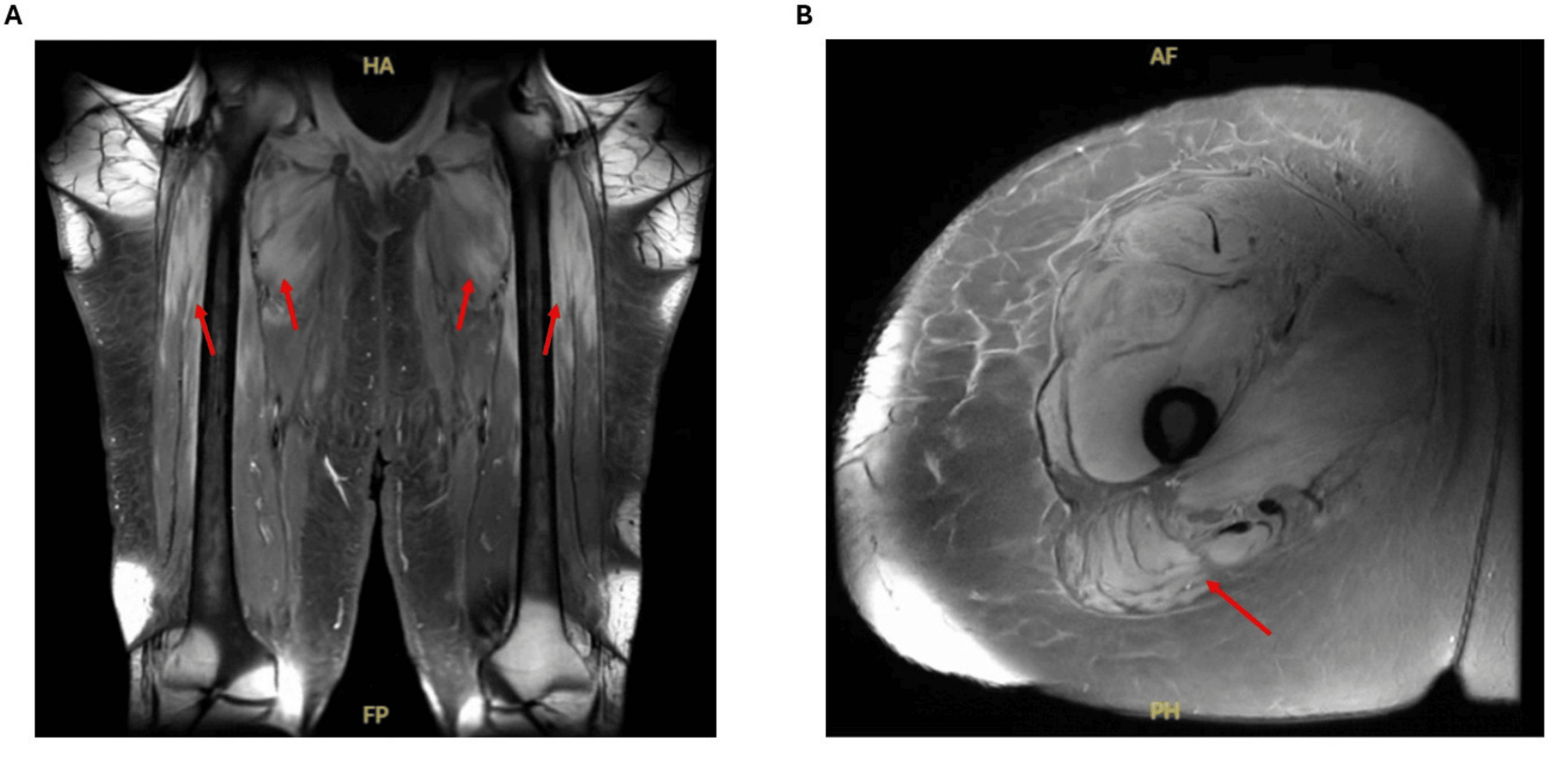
Coronal (A) and Axial (B) MRI Sequences Showing Diffuse Muscle Edema Involving the Anterior, Posterior, and Medial Compartments of the Proximal Thighs, Consistent with Active Inflammatory Myositis
